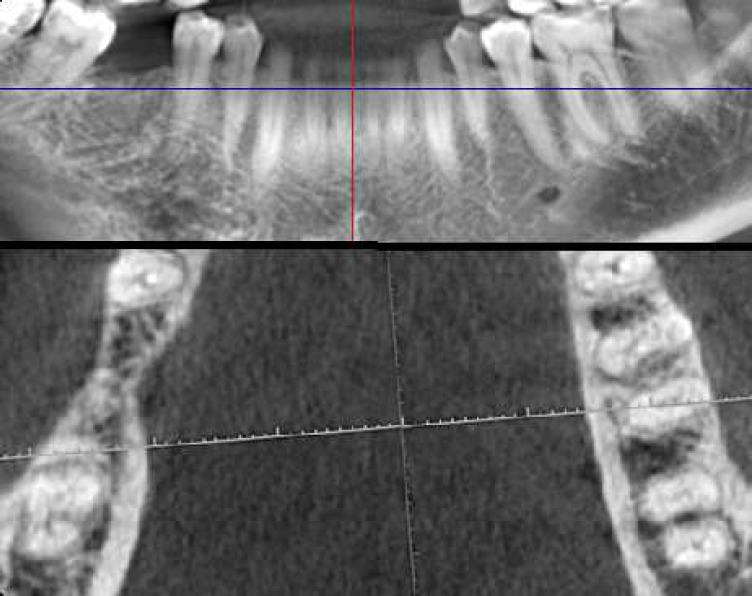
CBCT images of unilateral C-shaped mandibular second molar
